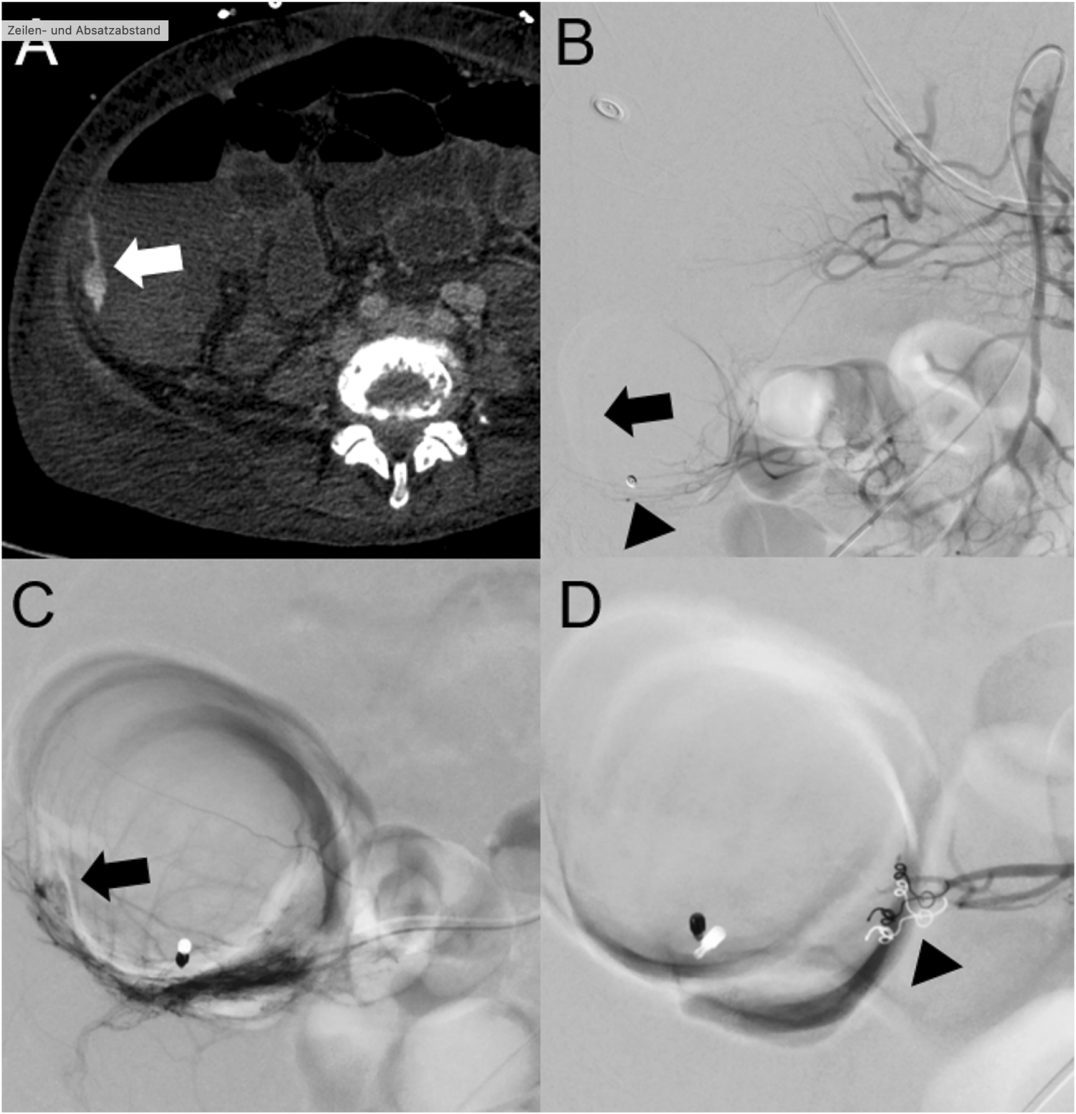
A A 62-year-old female patient with liver cirrhosis and active bleeding at the cecal pole with a clear extravasation (white arrow) on angio-CT. B Digital subtraction angiography showing a mesenteric image. The black arrow is pointing at the region of bleeding referring to the angio-CT (Black arrowhead: endoscopic clip). C Selective angiography with an extravasation. D Technically successful selective embolization with particles (Contour 355-510) and Coils (black arrowhead)
